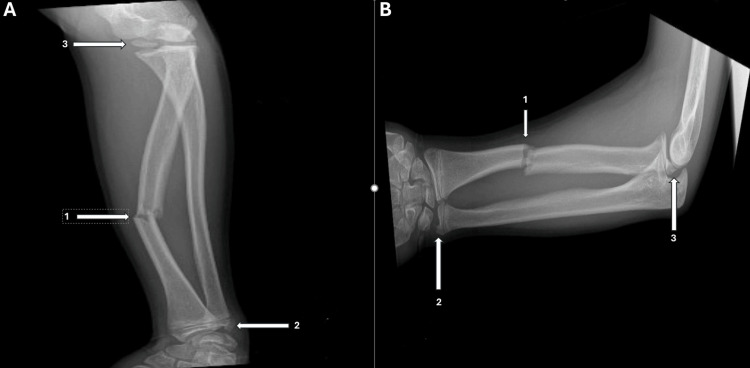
X-ray of the forearm, elbow, and wrist (anteroposterior and lateral view) A: Anteroposterior X-ray showing a fracture with dorsal angulation of the radial diaphysis (arrow 1) associated with avulsion of the ulnar styloid (arrow 2) and avulsion dislocation of the epiphyseal nucleus of the epicondyle (arrow 3). B: Lateral X-ray showing a fracture with dorsal angulation of the radial diaphysis (arrow 1) associated with avulsion of the ulnar styloid (arrow 2) and avulsion dislocation of the epiphyseal nucleus of the epicondyle (arrow 3).
